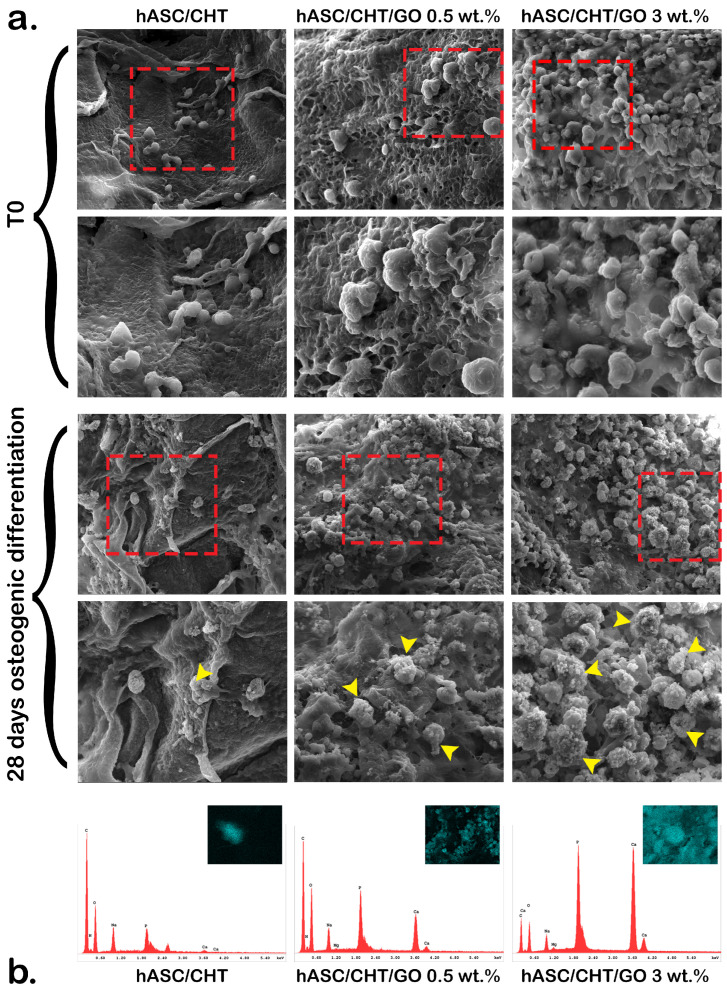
Cell distribution and phenotype in BC0.5–BC3 systems. (a) hASC distribution and morphology in the 3D structure of BC0.5–BC3 and the hASC/CHT reference bioconstruct (BC) before and after 28 days of osteogenic differentiation, assessed by SEM; the red box marks the area enlarged below each image and the yellow arrows indicate mineralized deposits in the extracellular matrix (ECM) which was further characterized by EDAX; (b) the composition of extracellular matrix secreted by cells after 28 days of osteogenic differentiation, as revealed by energy-dispersive X-ray analysis (EDAX) analysis.
